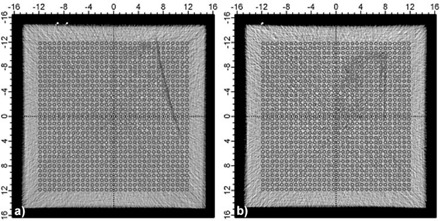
CT images of: a) Sample A and b) Sample B, showing representative density variations relative to the positions of the individual ion chambers in the 2D array.
